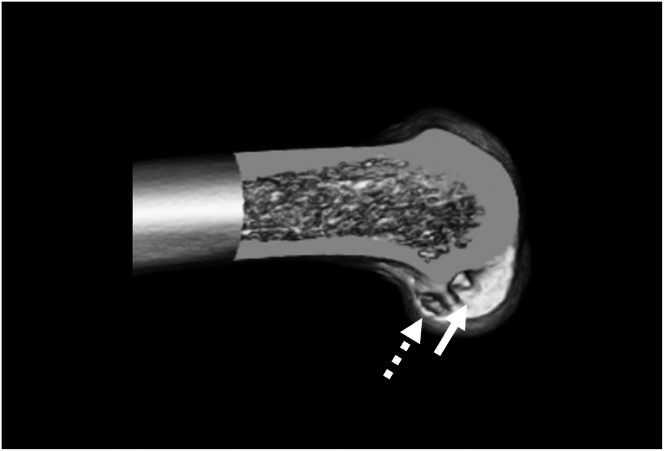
Postoperative 3-dimensional computed tomography scan of the patient’s left femur showing a new oval-shaped tunnel (white dashed arrow) deep and inferior to the initial tunnel (white solid arrow). The new tunnel is now located over the native anterior cruciate ligament footprint without tunnel overlap.
